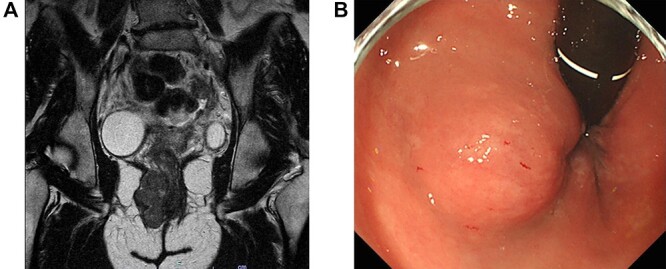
(A) Magnetic resonance imaging of the pelvis (sagittal section) demonstrates a 4.6 × 2.8-cm tumor in the right perineal area. (B) Proctoscopy reveals a submucosal tumor near the right wall of the anal canal.
